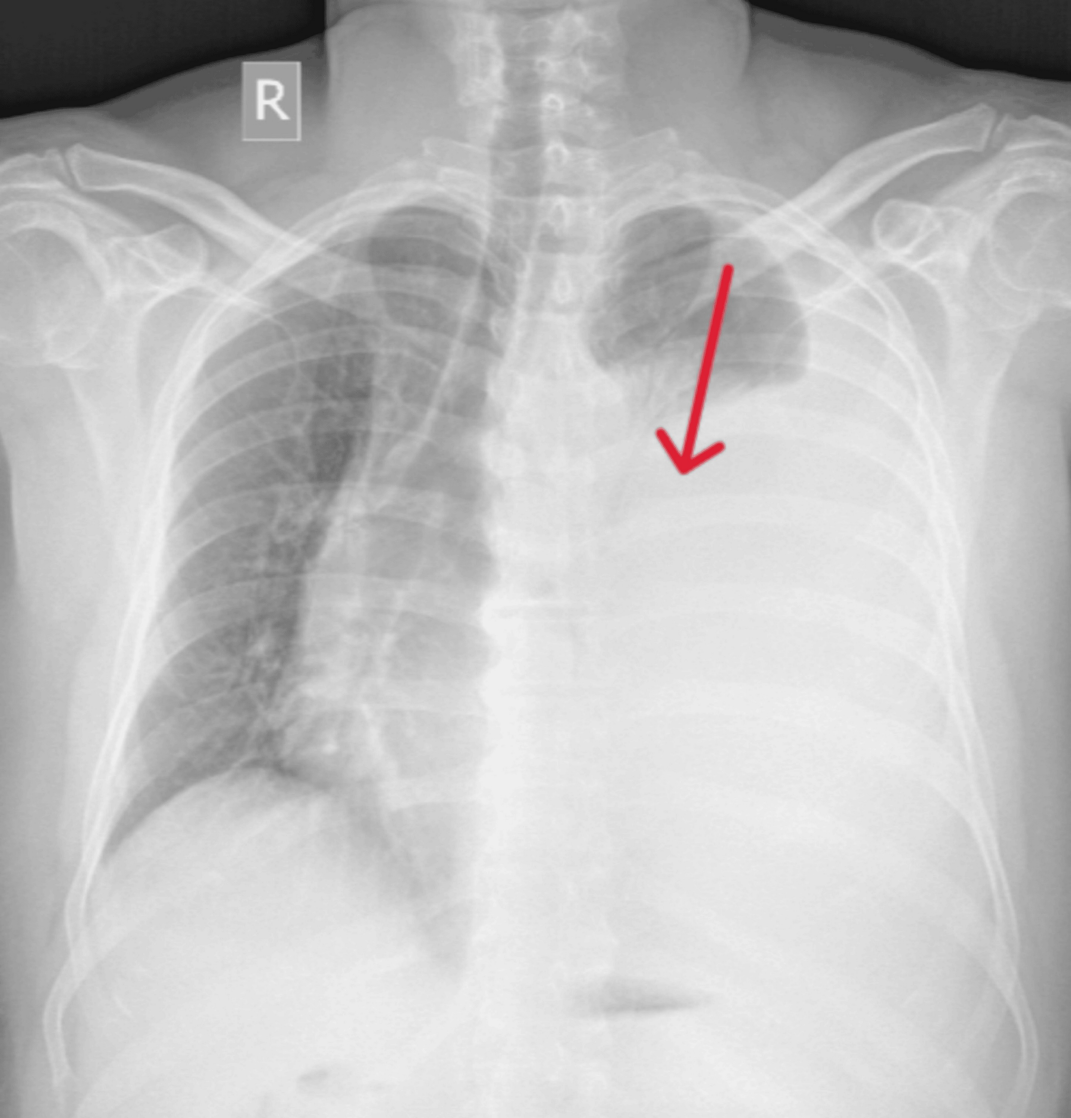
Chest X-ray showing a large left-sided pleural effusion (red arrow).
